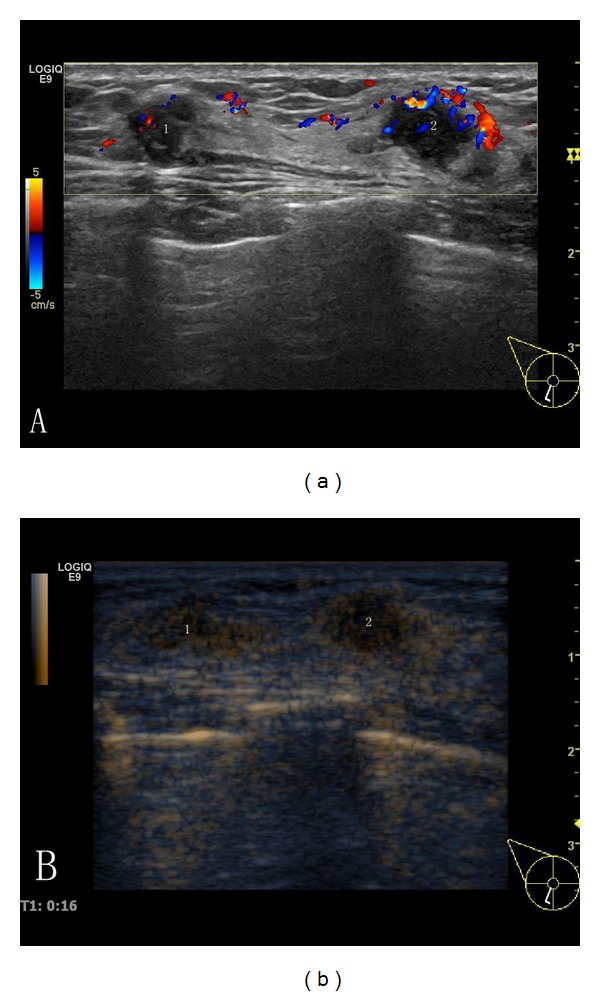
A sonogram of a right breast from a 57-year-old woman is shown. (a) There were two solid hypoechoic lesions at 7 o'clock in the right breast (lesion 1: 6 × 5 mm and lesion 2: 9 × 7 mm), with a circumscribed margin and defined, irregular shape, with a heterogeneous internal echo and nodular posterior echo enhancement. CDFI (color Doppler flow imaging): rich color flow signals were clearly visible in the nodule and its surrounding. Ultrasound diagnosis: BI-RADS 4c. CEUS (b): in the early arterial phase, nodules within its surrounding with significantly heterogeneous concentric enhancement can be seen. The range of strengthening was greater than the range that was in US. CEUS diagnosis: BI-RADS 5. Pathology: invasive ductal breast carcinoma.
